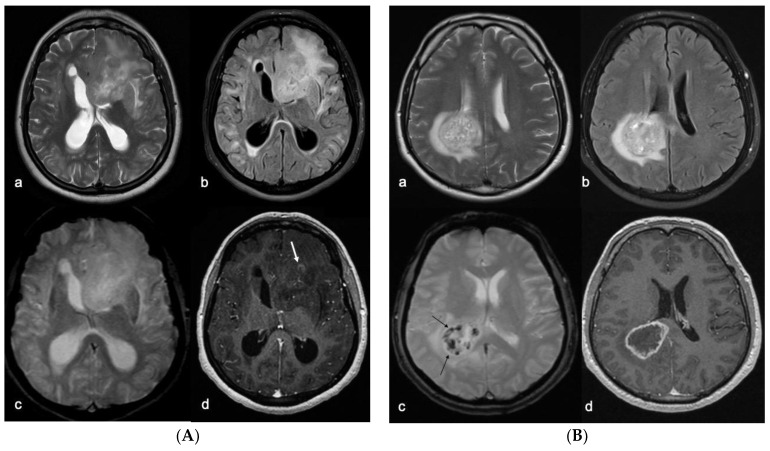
(A) Left frontal GBM with typical imaging findings of MGMT methylated (inactive) tumors. Despite the size of the lesion and its heterogeneous signal on the T2w fast spin echo (a) and T2w FLAIR images (b), there are no calcifications or intratumoral bleedings on the T2*w gradient echo images (c) and only a small irregular area of contrast enhancement appreciable after i.v. administration of contrast medium on the T1w pulse sequences (arrow in (d)). (B) Right GBM with typical imaging findings of the MGMT not-methylated subtype. The lesion is deeply localized in the fronto-parietal periventricular zone, with a heterogeneous signal on the T2w fast spin echo (a) and T2w FLAIR images (b), calcifications and/or bleedings on the T2*w gradient echo images (arrows in (c)), with a typical ring enhancement after i.v. administration of contrast medium on the T1w pulse sequences (d) that delimits a central area of intratumoral necrosis.
